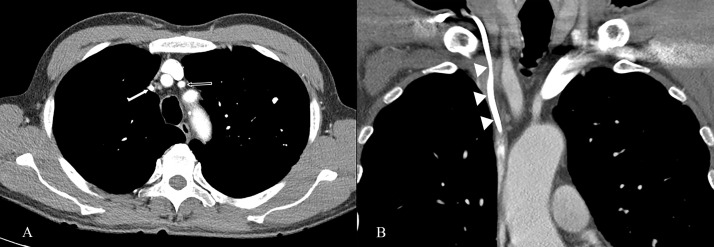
Representative images from a 66-year-old male patient diagnosed with sigmoid colon cancer who experienced CR-RIVS. a) Axial contrast-enhanced CT scan showing the diminished cross-sectional area of the right innominate vein (white arrow) compared to that of the left carotid artery (grey arrow). b): Contrast-enhanced CT scan in the coronal plane showing long segmental right innominate stenosis along the catheter (arrowheads). CR-RIVS, chemoport-related right innominate vein stenosis; CT, computed tomography.
